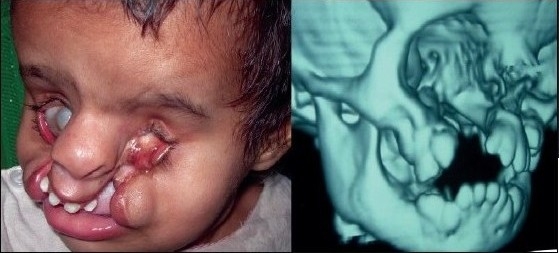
A case of bilateral Tessier cleft type-4 presented to us at one year of age. The infra-orbital margin & floor of the orbit were deficient (see CT Scan) & the globe was displaced inferiorly to such an extent that the upper eyelid was insufficient to cover the cornea. The cornea was already damaged
